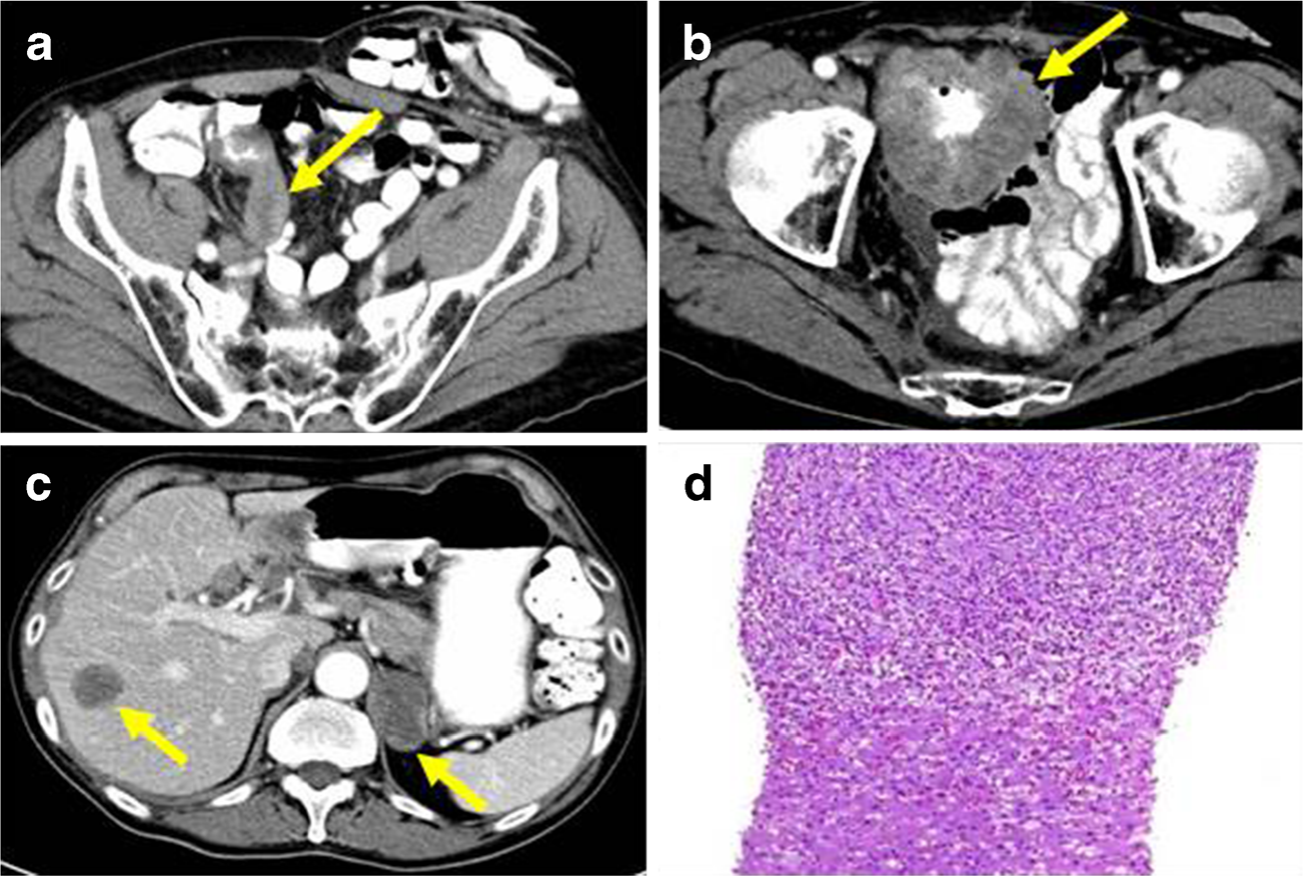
PATHOLOGY: metastatic IMT of the appendix. A 63-year-old man with antecedents of left hemicolectomy due to colon cancer. a Axial reformation of contrast-enhanced CT on portal phase acquired seven years after partial colectomy. An appendicular, irregular, solid mass with slight enhancement was identified (*yellow arrow*). Due to its radiological features, the lesion was diagnosed of appendicular carcinoma. The patient did not perform surgery. b Axial reformation of contrast-enhanced CT on portal phase performed 8 months later showed a significant growth of the appendicular mass with extension and infiltration of the adjacent small bowel loops. c Distant lymphadenopathy and liver metastases were present
